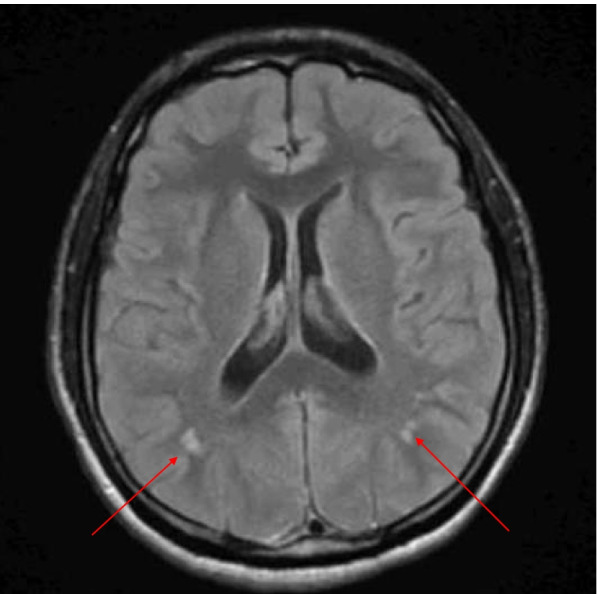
Axial T2 FLAIR sequence demonstrating T2 signal prolongation along the gray, white junction bilaterally
